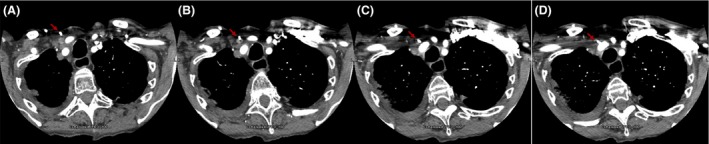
CT angiogram transverse views A‐D. Red arrow indicating the Port‐A‐Cath entering the right subclavian artery.
